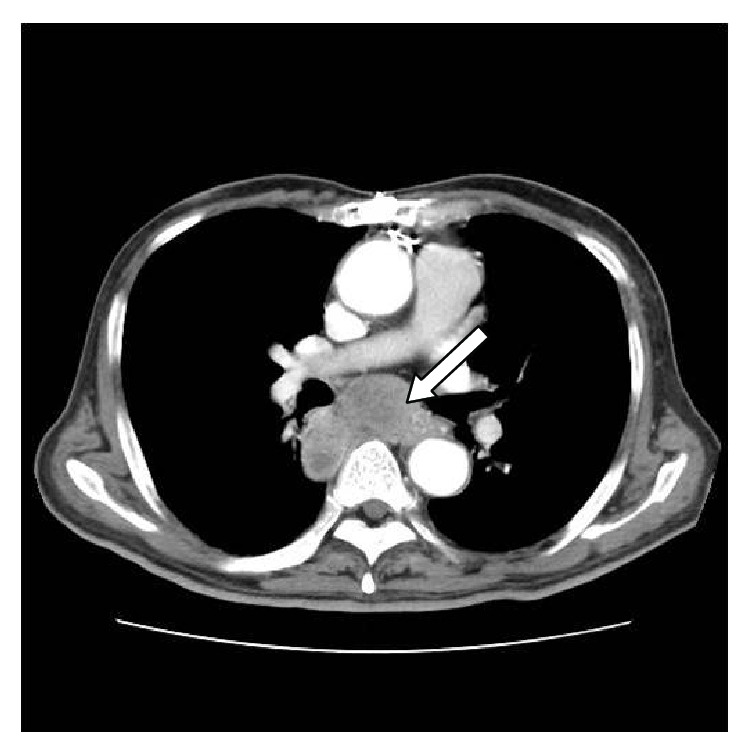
Chest computed tomography scan shows the multiple metastatic lymph nodes enlargement at subcarinal area (white arrow).
